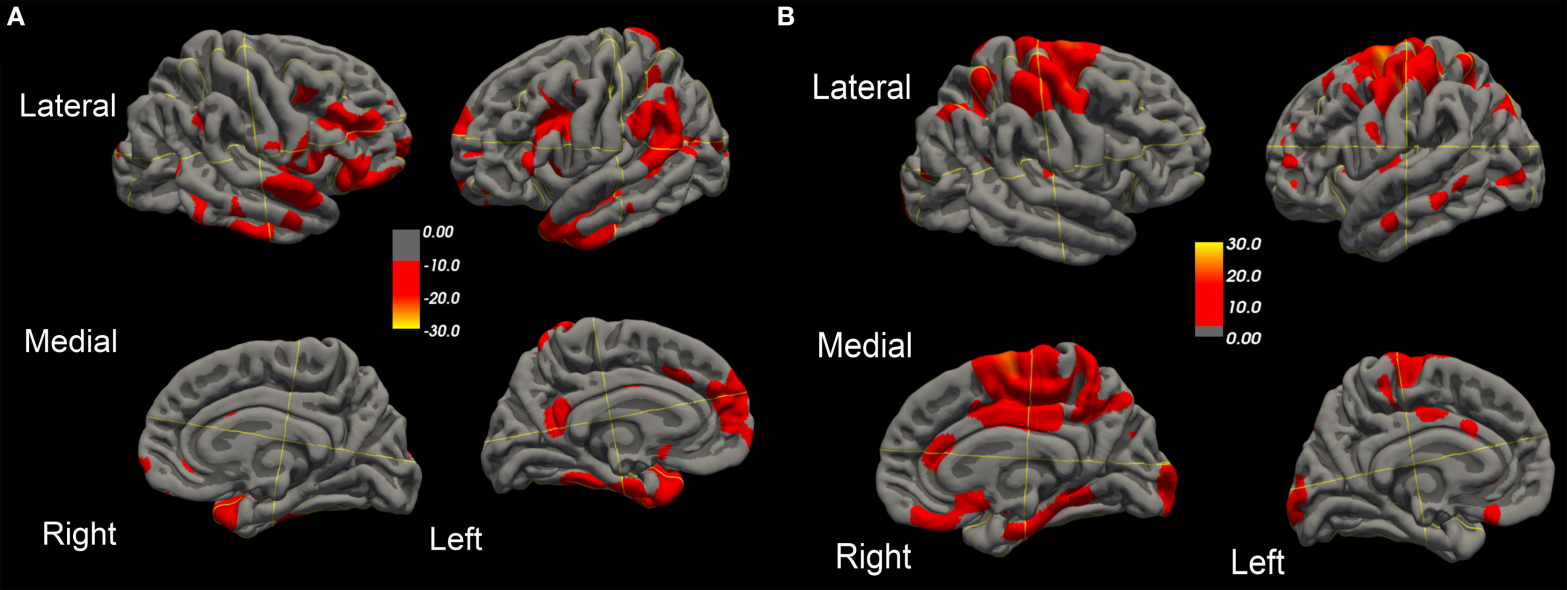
Surface-rendered MRI of right and left hemispheres show symmetrized percent change (SPC) values of cortical thickness decrease (cortical thinning) (A) or increase (cortical thickening) (B) in the ALS-FTD patient over 20.4 months.
